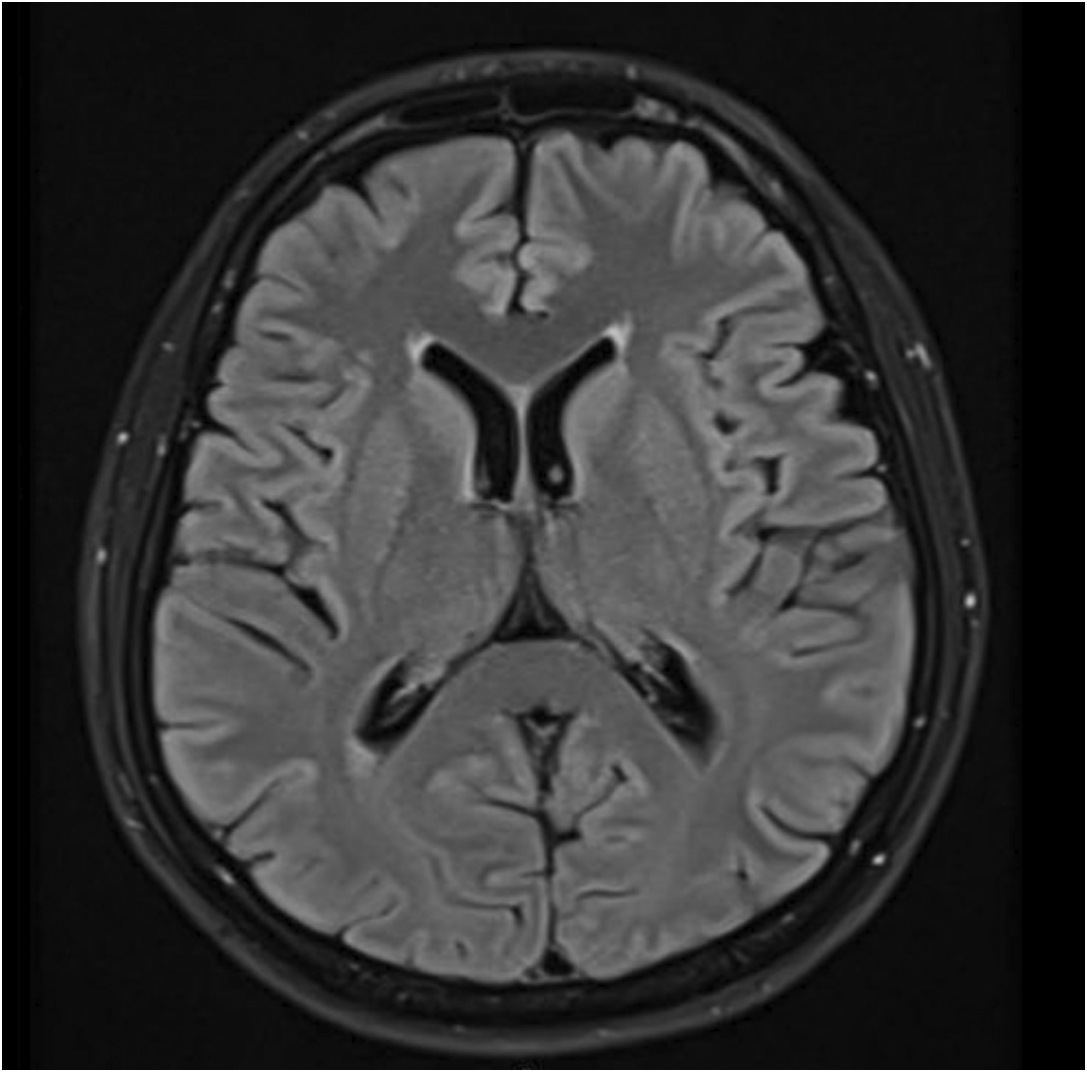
Initial MRI head showing normal study
